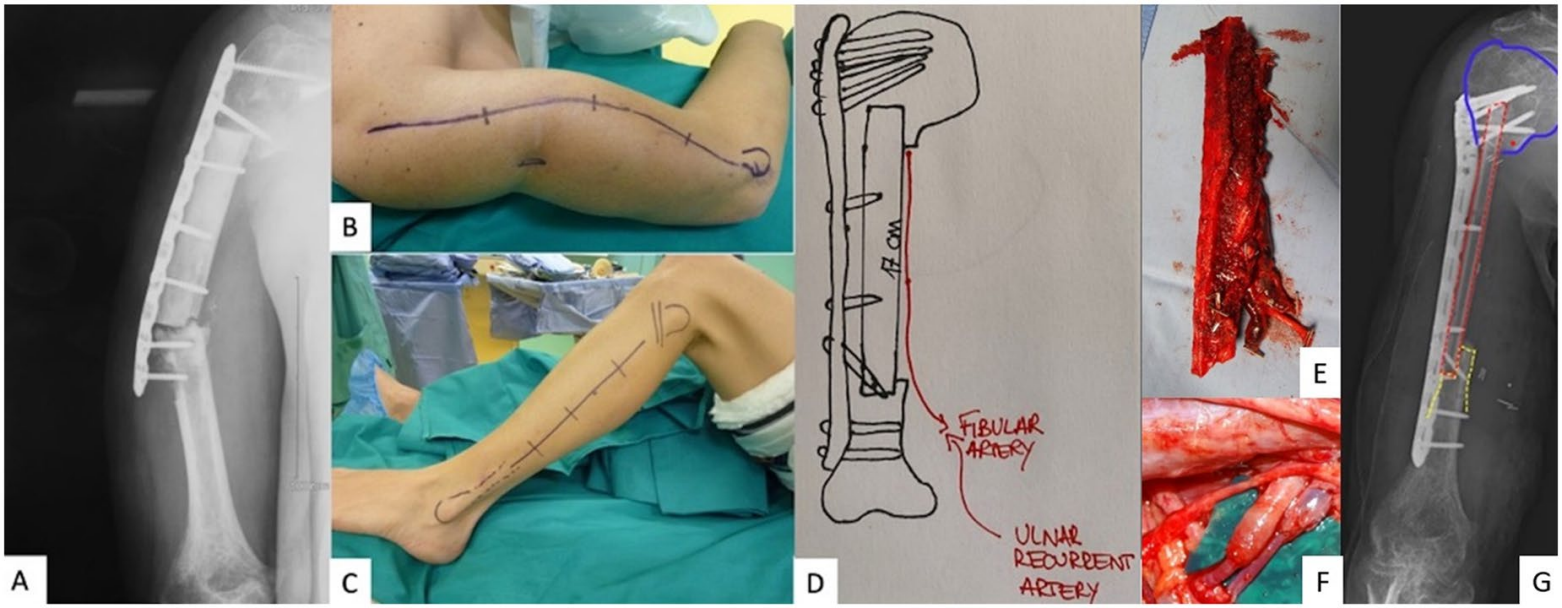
(Case 2). A. X-ray exams 11 months after surgery showing allograft non-union and hardware breakage. B. Clinical deformity of the right arm. C. Clinical picture of donor site for FVFG harvesting. D. Image of preoperative planning. E. FVFG harvested with peroneal vessels. F. Microvascular anastomosis between peroneal vessels and ulnar recurrent vessels. G. Postoperative X-ray. The humeral head is surrounded by blue dashed line. FVFG is surrounded by red dashed line. Step-cut osteotomy is underlined by yellow dashed line.
